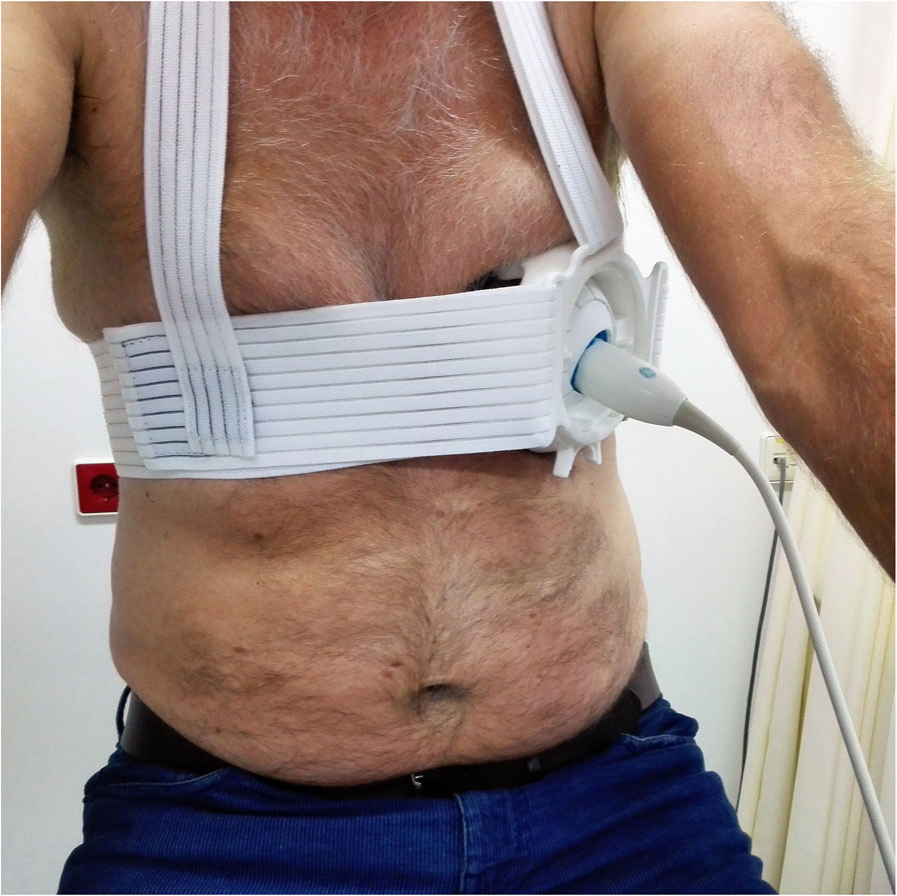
Apical fixation of the Probefix for echocardiography. The ultrasound probe (M5Sc, GE Healthcare, Milwaukee, USA) is fixated to the patient’s chest with the Probefix. The patient is sitting upright on the bicycle ergometer. First the optimal apical four chamber position is searched for without the fixator. Next, the probe is placed in the Probefix, which is then fixated to the patient by attaching the chest straps
